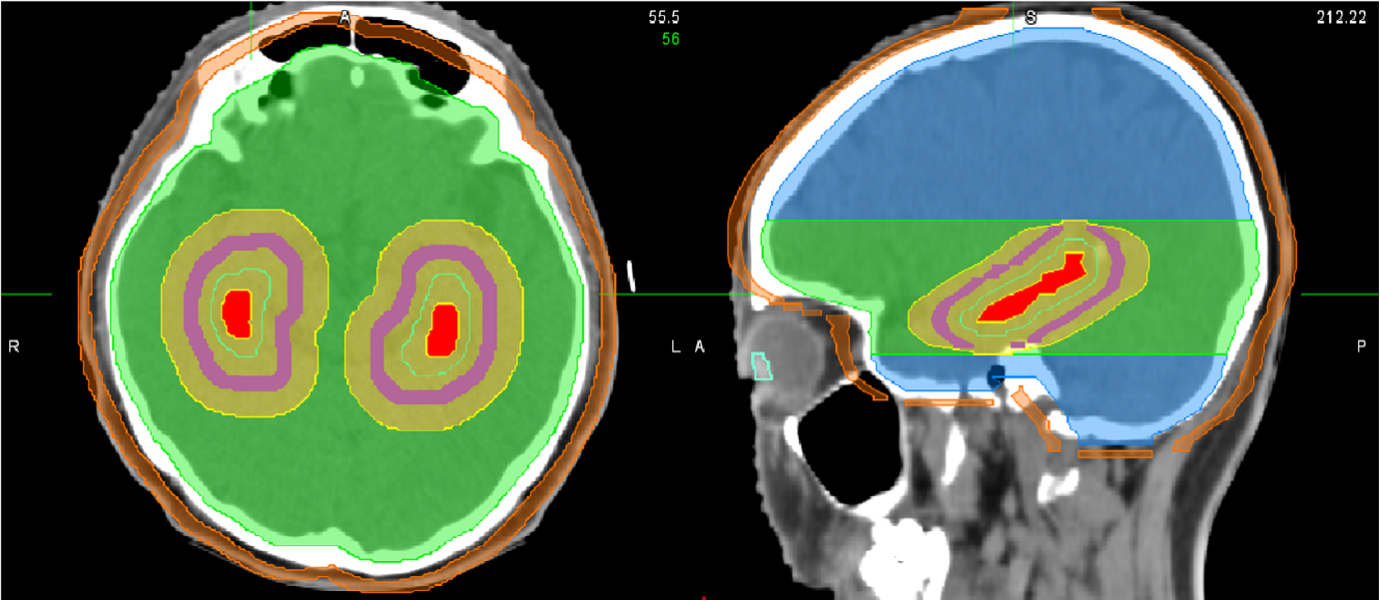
Axial and sagittal CT images showing hippocampus (red), hippocampus PRV (cyan line), PTV_plan1 (green), PTV_plan2 (blue), dose decline region (yellow), ring PRV (pink), and ring PTV (orange).
